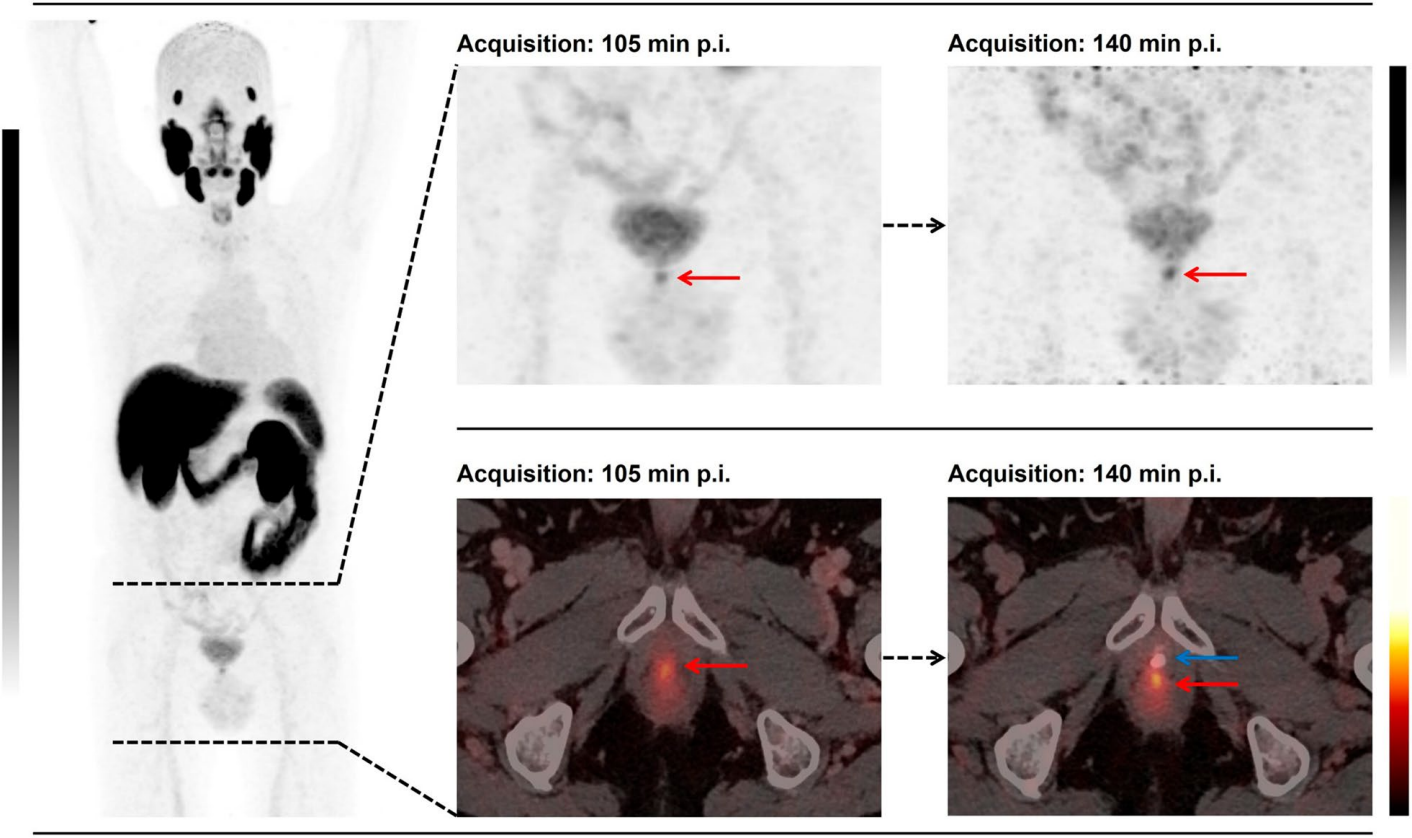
The juxtaposition of focal [^18^F]PSMA-1007 uptake (red arrow) to the urethra (blue arrow) complicates the accurate discrimination from residual radiourine in the urethra. With increasing tracer uptake of the lesion and detection of contrast agent in the urethra, an upgrading of PSMA-RADS, from 3 A to 5, could be reported
